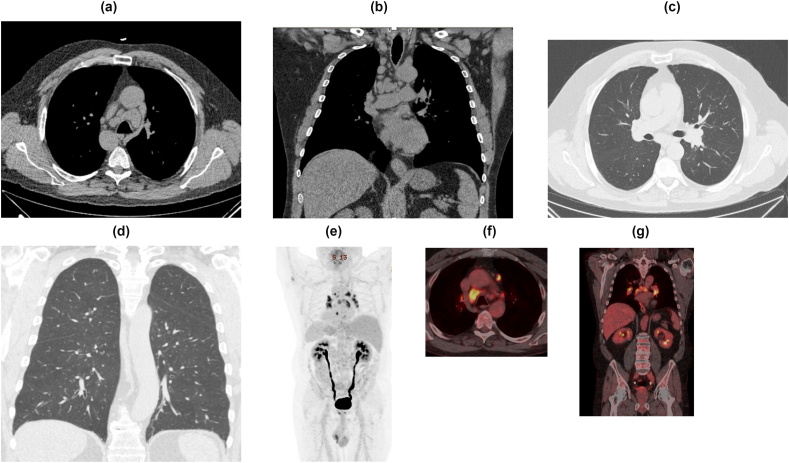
a, b, c, d, e, f, g: CT images obtained in July 2019. (a, b): Axial and Coronal CT images show numerous enlarged mediastinal and paratracheal lymph nodes (arrows). (c, d): Axial and Coronal CT images with lung window settings show normal lung parenchyma. (e,f, g): Corresponding PET-CT images with increased metabolic activity of the mediastinal and paratracheal lymph nodes.
